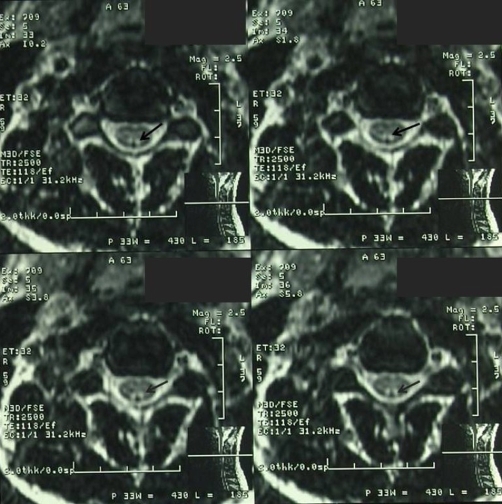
Increased signal intensity within the posterior column of the cervical spine on the axial T2 weighted MRI.
